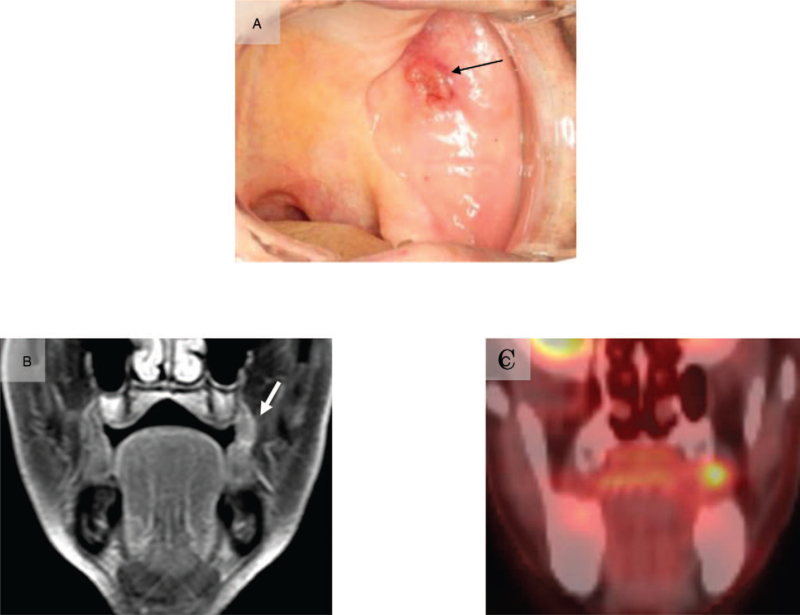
Clinical findings. An elastic-hard mass measuring 17 × 15 mm in size was observed on the left buccal mucosa (A). The mass exhibited moderate intensity on contrast T1-weighted magnetic resonance imaging, and advancement to the buccinator muscle was noted (B). On fluorodeoxyglucose-positron emission tomography (FDG-PET) with an SUVmax of 4.6, FDG accumulation was detected in the left buccal mucosa (C).
